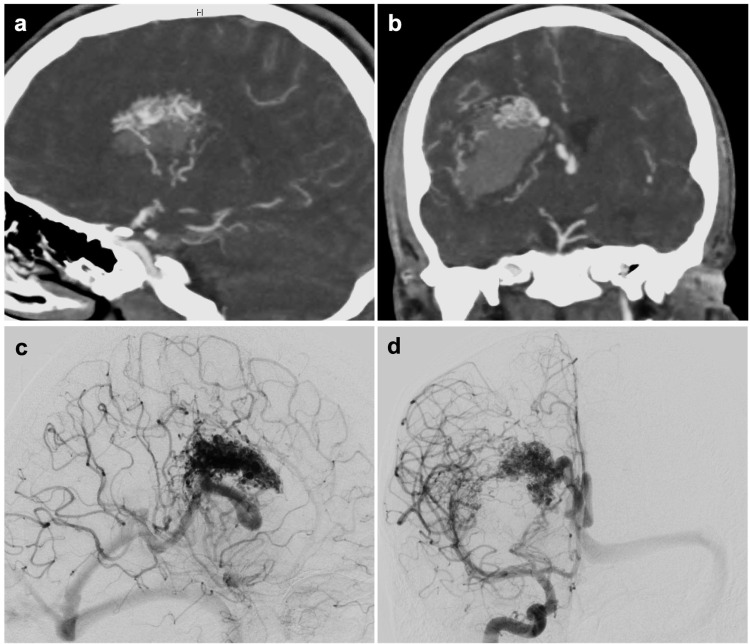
Diagnostic imaging of right frontal AVM. CT angiography of the head in the sagittal (A) and coronal (B) planes showing the right frontal AVM that is adjacent to the basal ganglia and internal capsule. (C, D) Diagnostic angiogram demonstrating AVM nidus and lenticulostriate artery feeders. AVM, arteriovenous malformation.
